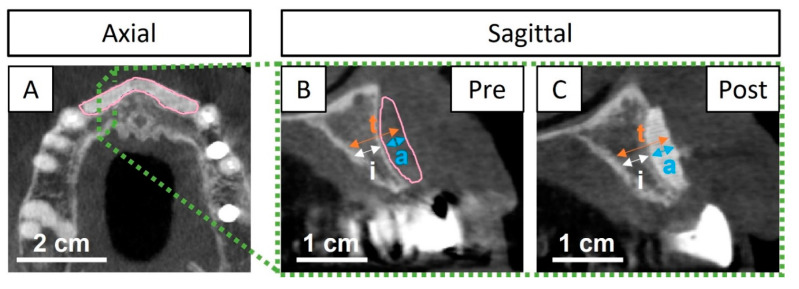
Representative CBCT images to show how the measurements of the bone thickness were performed. The pink line indicates the contour of the pre-designed graft, and the green lines indicate the position and projection where measurements were performed. (A) Axial projection with pre- and post-surgery CBCT images superimposed; (B,C) a sagittal projection indicates how the different measurements were obtained, (B) pre-surgery (i.e., initial bone and pink line indicating planned augmentation), (C) post-surgery (i.e., initial bone and grafted area): i = initial bone thickness, a = bone thickness augmentation (i.e., gained bone) and t = total bone thickness; t = (i + a).
